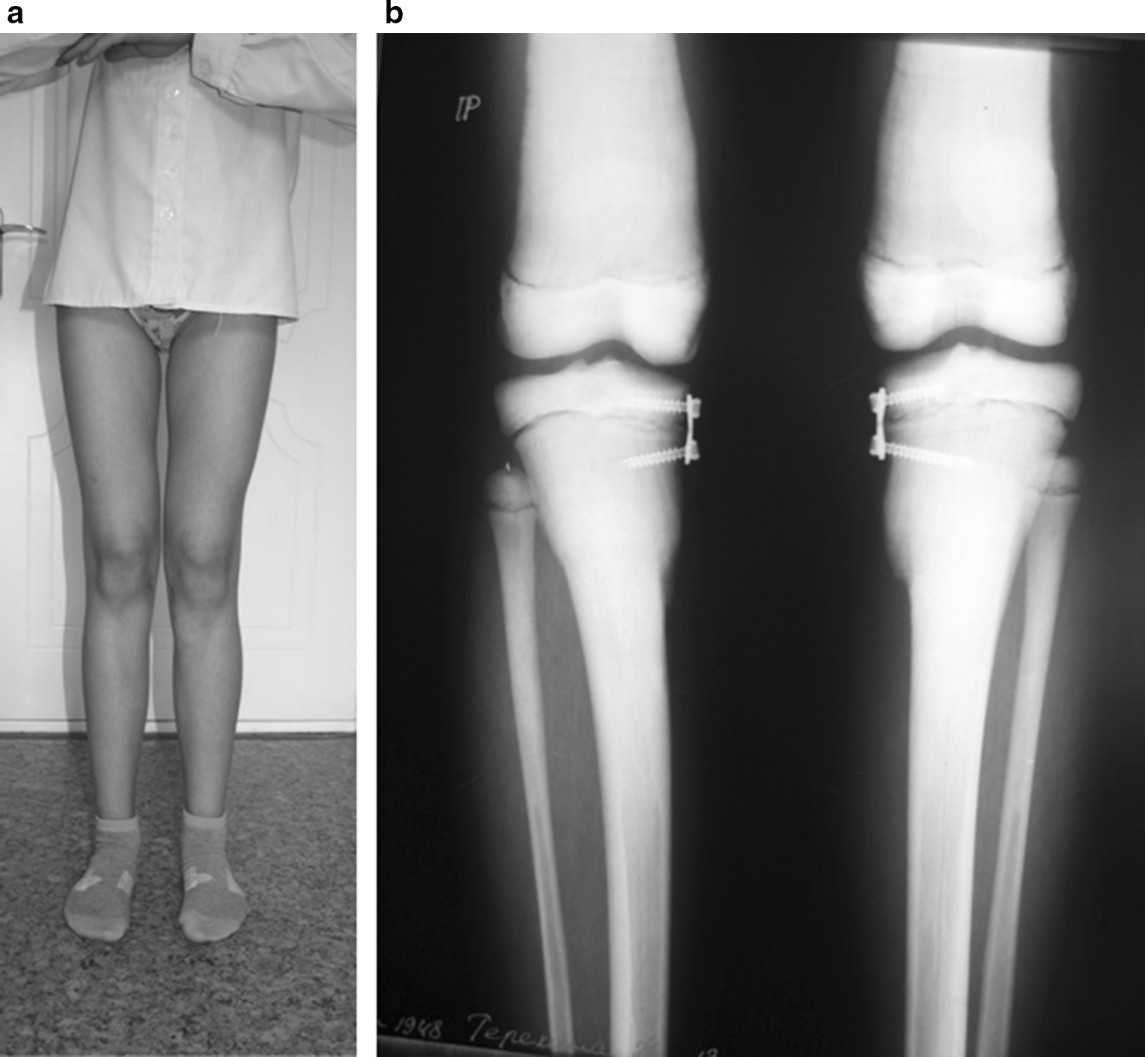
During treatment: a lower limb aspect in 9 months after surgery; b AP radiographs of lower limbs in 12 months after eight-plate implant
